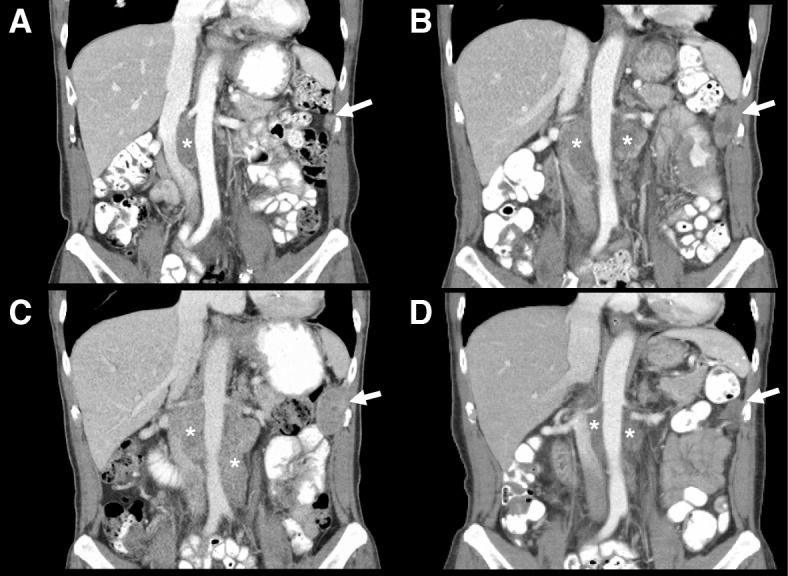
Representative coronal reconstruction from CT scans obtained throughout the clinical course. (*) indicate retroperitoneal lymphadenopathy and white arrows indicate peritoneal metastases. a CT scan from initial staging (prior to neo-adjuvant chemotherapy). b Progressive disease while on pemetrexed. c Further progression on paclitaxel. d Significant reduction in tumor burden following third dose of pembrolizumab
